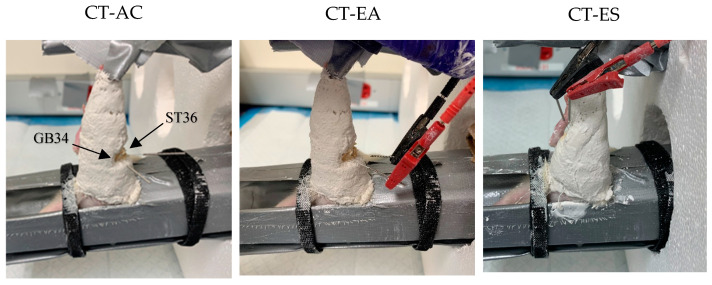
Stomach 36 (ST36) and Gallbladder 34 (GB34) needle position. CT-AC: acupuncture, CT-EA: electro-acupuncture, and CT-ES: electrical stimulation.
